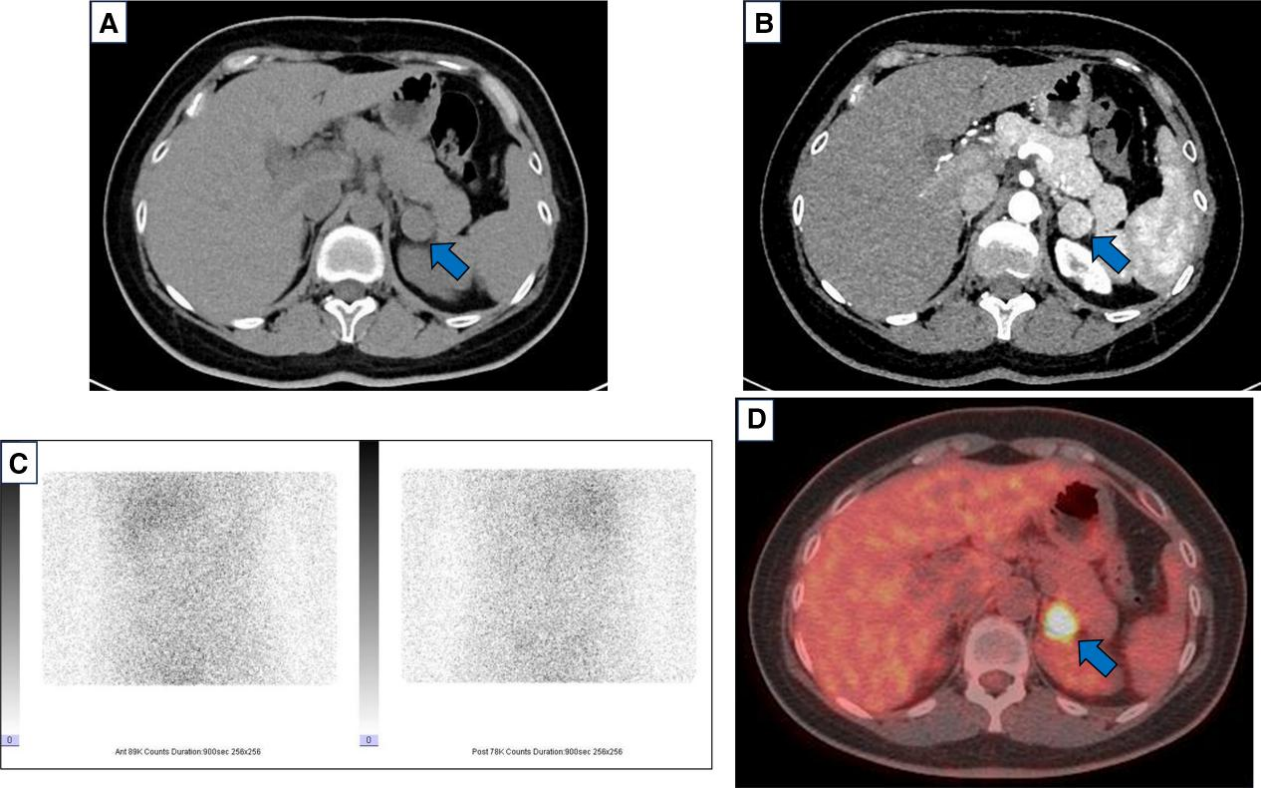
Radiological imaging of the left adrenal tumor. (A) Unenhanced computed tomography (CT) image showing a left adrenal tumor approximately 2 cm in diameter with relatively high density (mean Hounsfield units, 44), (B) Contrast-enhanced CT image showing heterogeneous contrast enhancement of the tumor, (C) ^131^I-adosterol scintigraphy image showing no enhanced isotope accumulation in the tumor, and (D) ^18^F-fluorodeoxyglucose positron emission tomography/CT image showing strong accumulation with maximum standardized uptake value 14.9 at the tumor.
